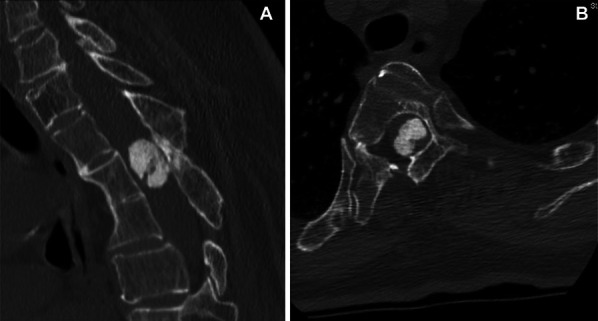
Cervico-thoracic computed tomography scan, using bone algorithm, showing the intradural round calcified mass occupying almost the entire spinal canal on A, the sagittal and B, axial plane.
